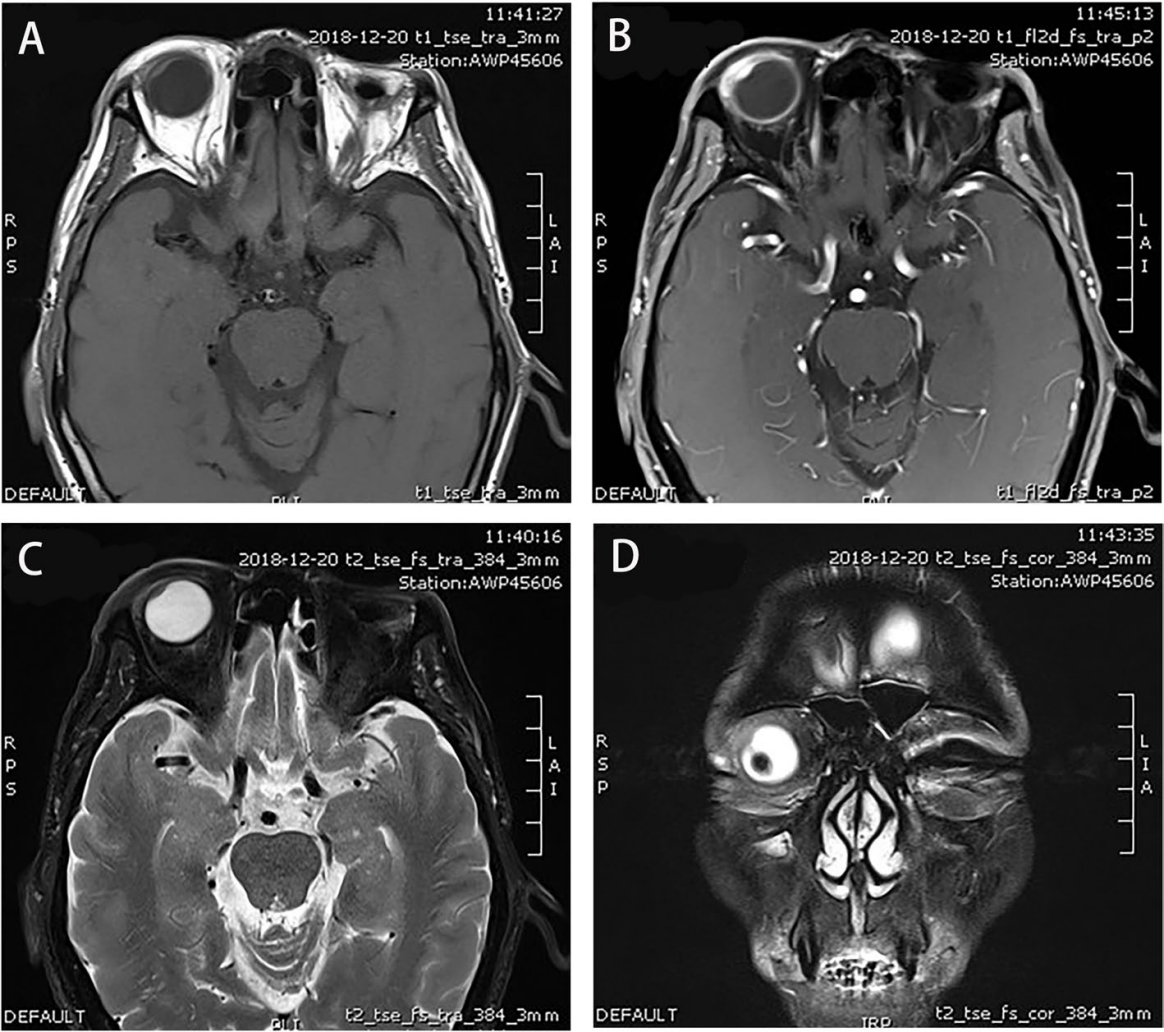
Magnetic resonance imaging (MRI) images of the right orbit at the patient’s second visit. A The lesion located in the ciliary body region of the right eye appeared isointense or slightly hyperintense on T1-weighted image (T1WI). B In T1WI post gadolinium enhancement MRI, the contrast enhanced scan showed enhancement in the lesion on T1-f12d fat suppression (fs). C and D The lesion located in the ciliary body region of the right eye appeared hypointense on T2-weighted image (T2WI) (C: T2-tse-fs-tra; D: T2-tse-fs-cor)
